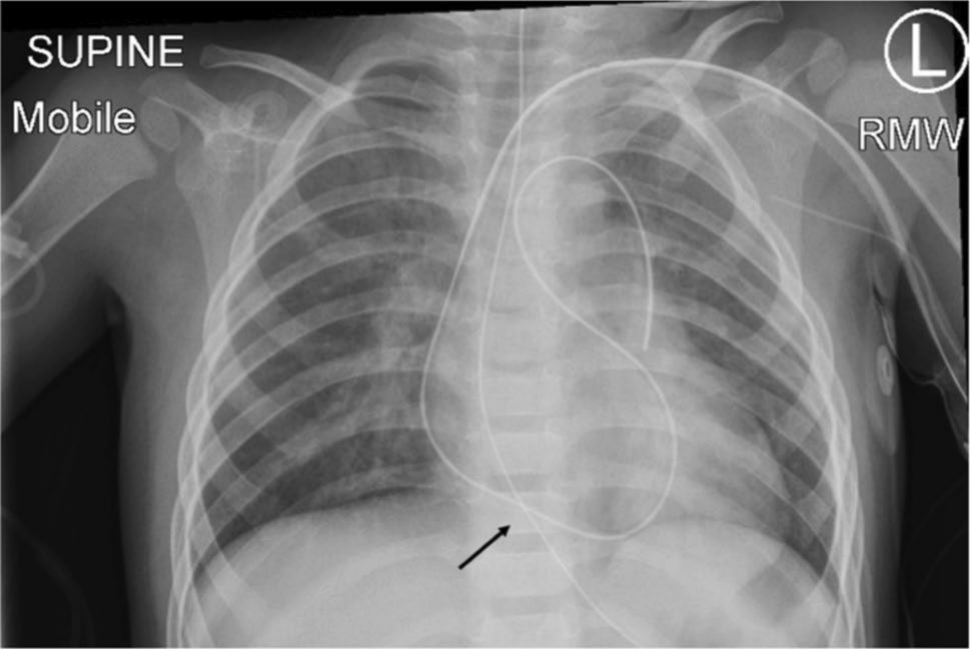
Chest X-ray showing loop of non-luminal channeled drain ensuring drainage of retro-cardiac space (black arrow)
